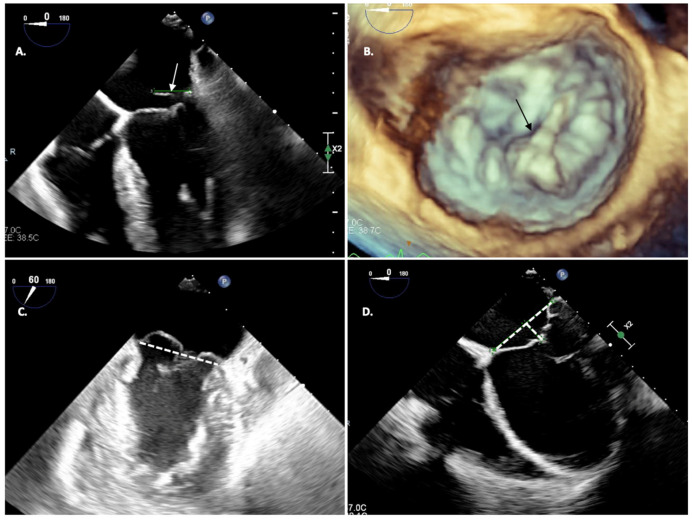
Echocardiographic evaluation of mitral regurgitation etiology; (A) PMV leaflet flail (white arrow); (B) PMV leaflet flail in 3D view (black arrow); (C) Bi-leaflet prolapse in Barlow disease (atrio-ventricular junction represented by white dashed line); (D) Tenting area in Secondary MR.
